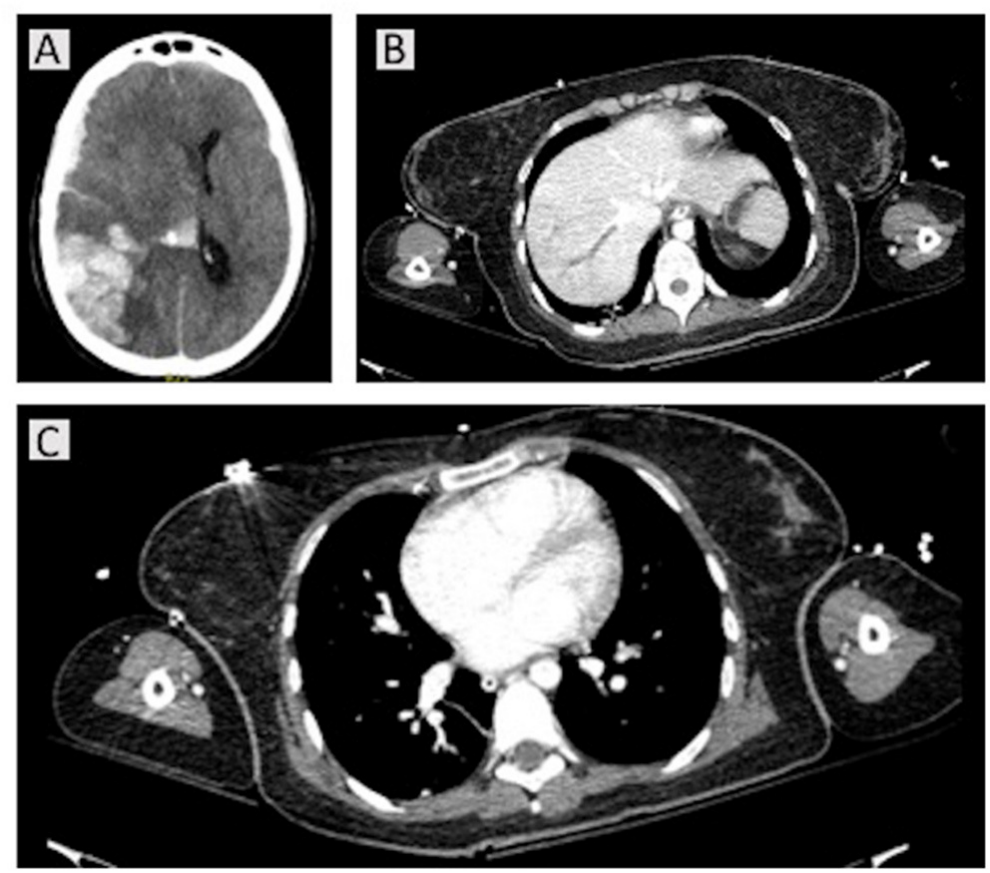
Computed tomography (CT) scans for case 1. A non-contrast brain CT and angio-CT were immediately assessed on hospital admission, showing right temporo-parieto-occipital intraparenchymal hemorrhage with intraventricular hemorrhage, fronto-parietal subdural hematoma, with homolateral fronto-temporal subarachnoid hemorrhage. She presented with intracranial hypertension and a 15-mm midline shift, with uncal, transtentorial, and cerebellar herniations into foramen magnum (A). The homolateral transverse and sigmoid sinuses were completely obliterated, as well as the jugular gulf; the sagittal superior sinus was partially obliterated. The intracranial arteries were obstructed. At thoracic CT perfusion and abdominal scans, pulmonary embolism in the arterial ramifications for the left inferior lobe and increased hepatic volume with thrombosis of the suprahepatic veins were present (B,C).
